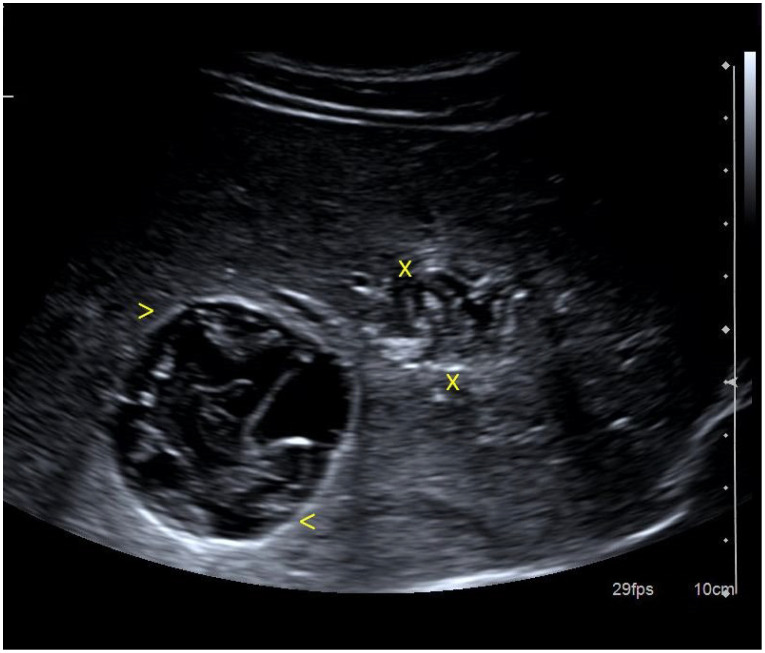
A 40-year-old female presents for consultation for right renal colic. Ultrasound revealed 2 cystic lesions (between markers x, and <) with thick walls and septa, situated in the right liver lobe. Anti Echinococcus granulosis antibodies positive. Typical aspect of hydatid cyst.
